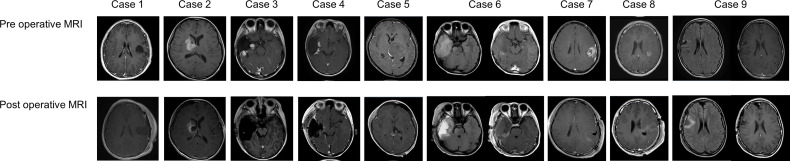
Magnetic resonance imaging findings for all enrolled cases.
